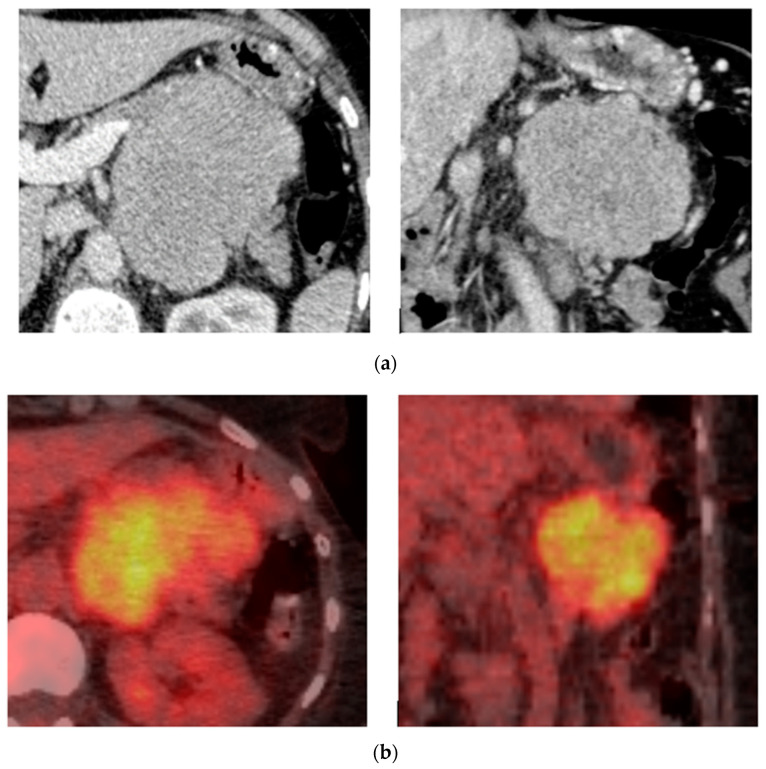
Biopsy-proven pancreas EP, case from our institution. (a) Post-contrast axial and coronal CT shows a well-defined homogenous lobular mass at the body of the pancreas; (b) Axial and coronal PET-CT shows increased FDG uptake in the pancreatic lesion.
